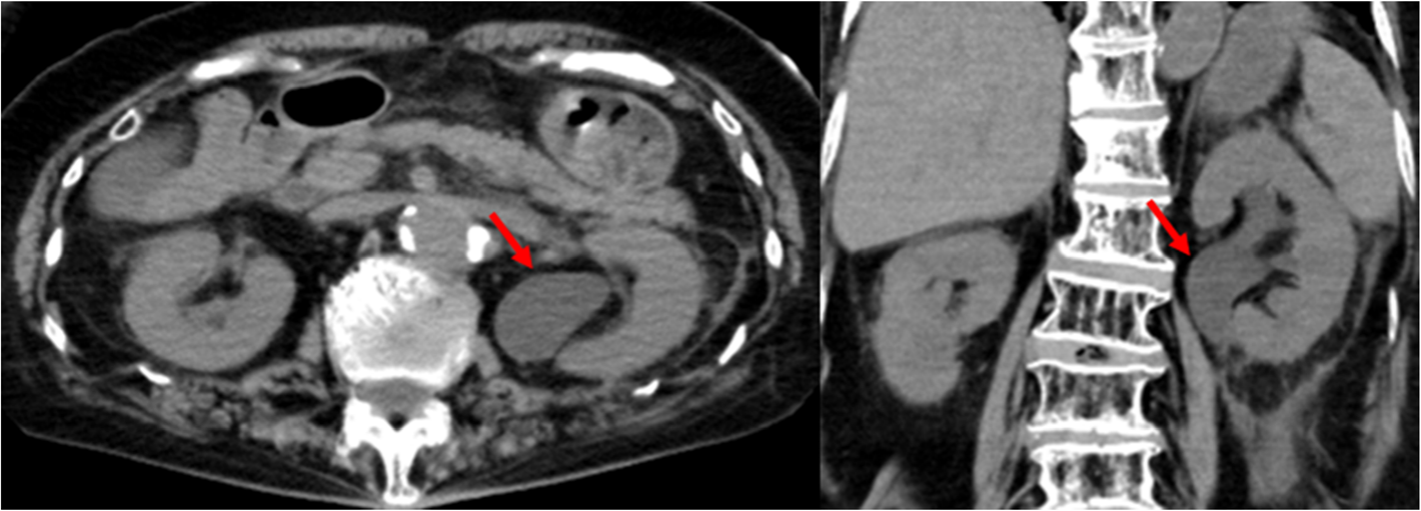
Computed tomography reveals left hydronephrosis with adipose tissue opacity around the left kidney
